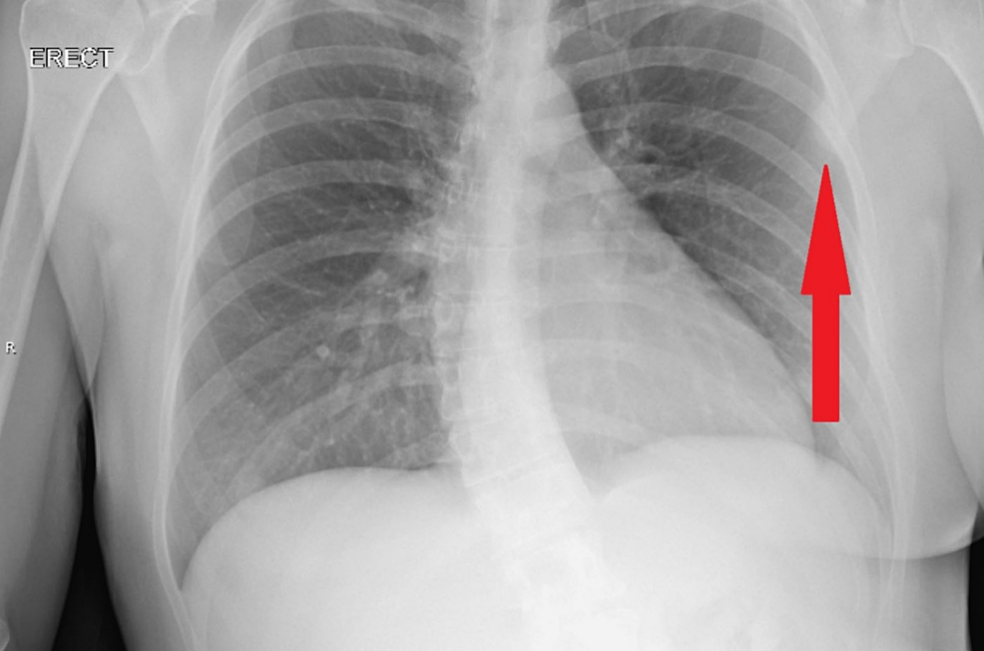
Anterior posterior radiograph pre-surgery show normal cardio-mediastinal silhouette. The pleural based nodule is seen above the arrow, in the left upper lobe adjacent to the third rib. There is no edema or airspace consolidation. Scoliosis noted in thoracolumbar spine.
